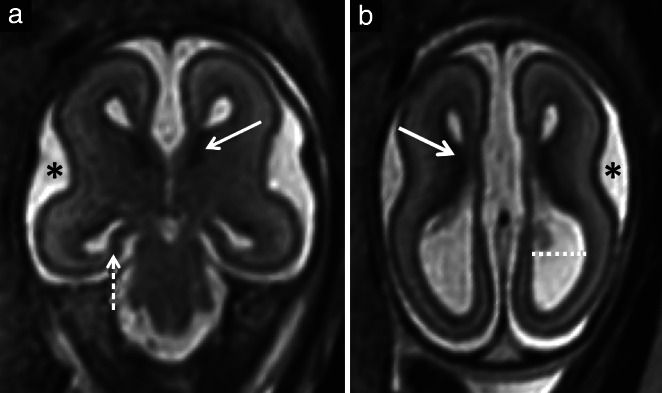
Example brain magnetic resonance images (MRI) in a fetus with isolated corpus callosal agenesis at 22 weeks' gestation that had a high MRI score. Coronal (a) and axial (b) T2‐weighted single shot fast‐spin echo images. There was delayed opercularization () (a,b), scoring 1 point, severely malrotated hippocampi that were almost completely flat (dashed arrow) (a), scoring 3 points, abnormal lamination with abnormally thick germinal matrix (solid arrows) (a,b), scoring 2 points, and moderate ventriculomegaly (dotted line) (b), scoring 1 point. (b) Unlike in Figure 3, lamination could be visualized adequately on T2‐weighted image.
